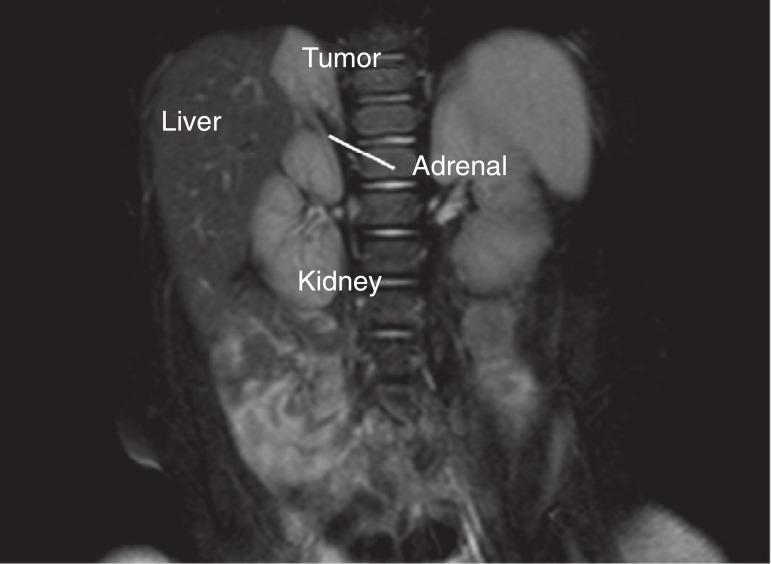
Close association between intra-abdominal extralobar pulmonary sequestration and right adrenal gland in magnetic resonance imaging (T2 images).
